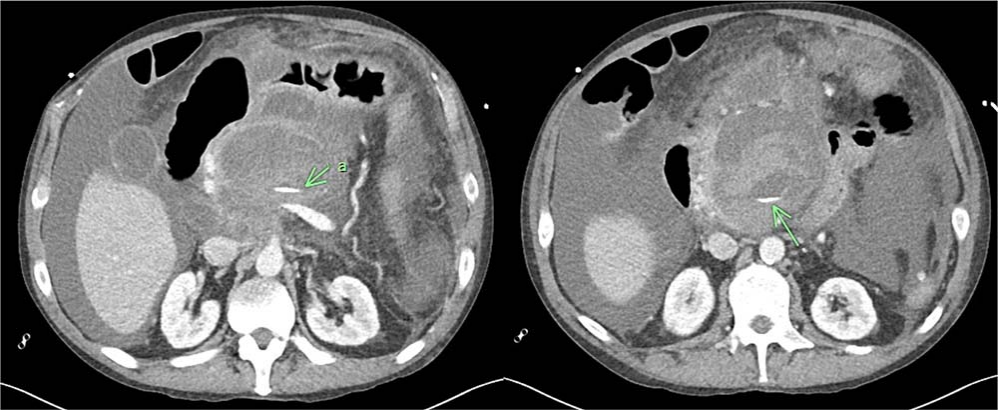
CT angiogram of abdomen axial view; contrast extravasation, indicating active bleeding within this peripancreatic collection.
